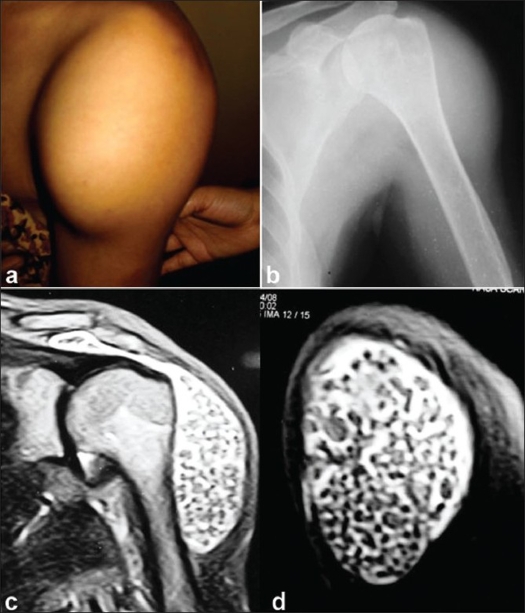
(a) A clinical photograph showing big swelling on the outer aspect of the left shoulder and left upper arm (b) X-ray left shoulder showing a soft tissue swelling in the deltoid region; no point/area of calcification visible in the swelling (c) MRI coronal section through the shoulder joint shows multiple nonossified loose bodies deep into the deltoid muscle with subacromial extension. No loose body below the rotator cuff (d) Another MRI sagittal section through the material of the swelling, showing a large number of loose bodies deep into the deltoid muscle; none is ossified
